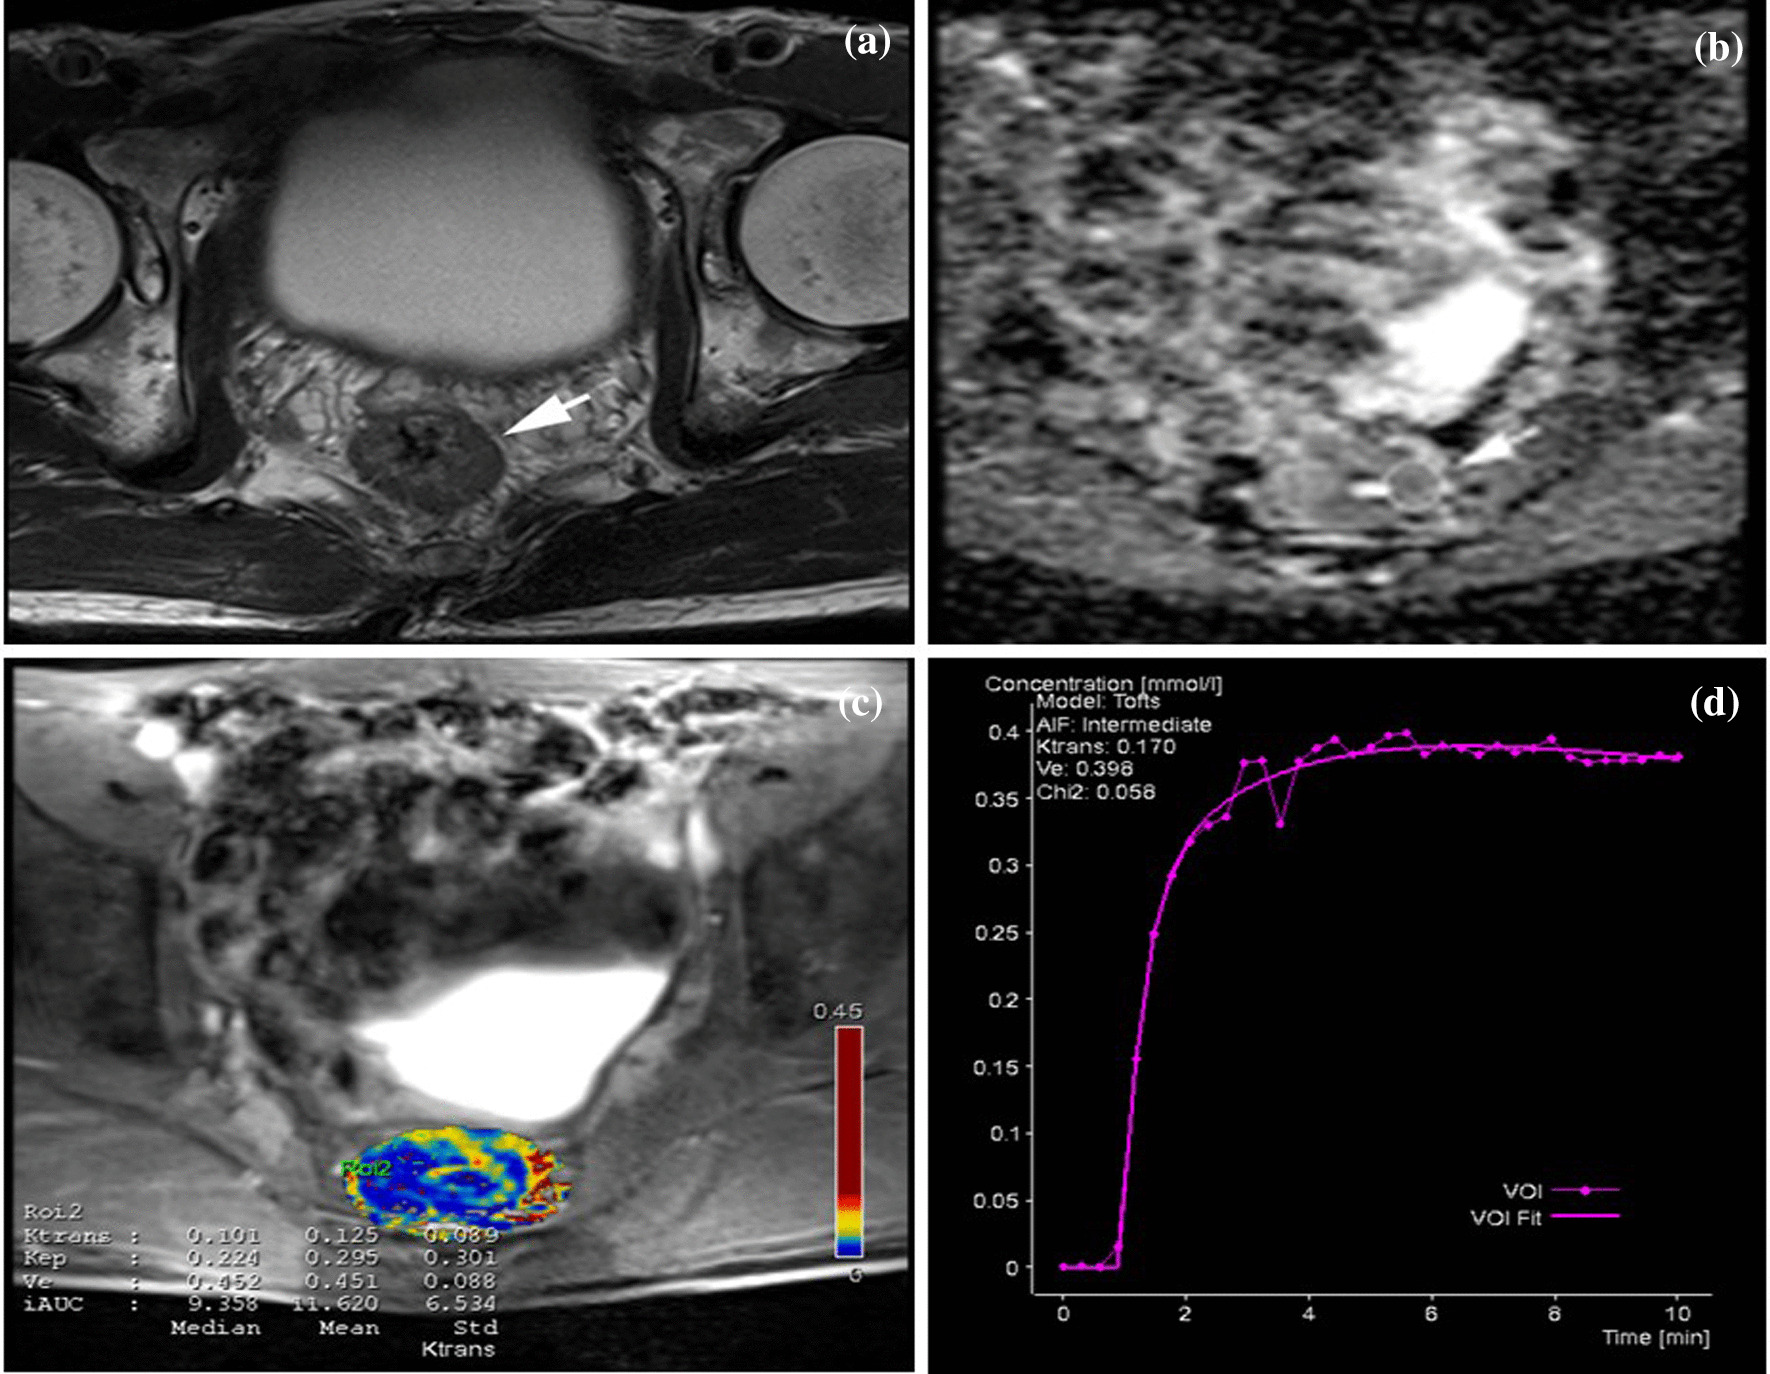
72-year-old man with Mr-detected extramural venous invasion (mrEMVI) negative rectal cancer. a High resolution T2WI (HR-T2WI) showed rectal wall thickening and no vessels adjacent to areas of tumor penetration. ADC (b) and perfusion (c) image of the rectal cancer segment showing ROI placement and DCE-MRI quantitative parameters values. d Time-signal intensity curve was platform curve
